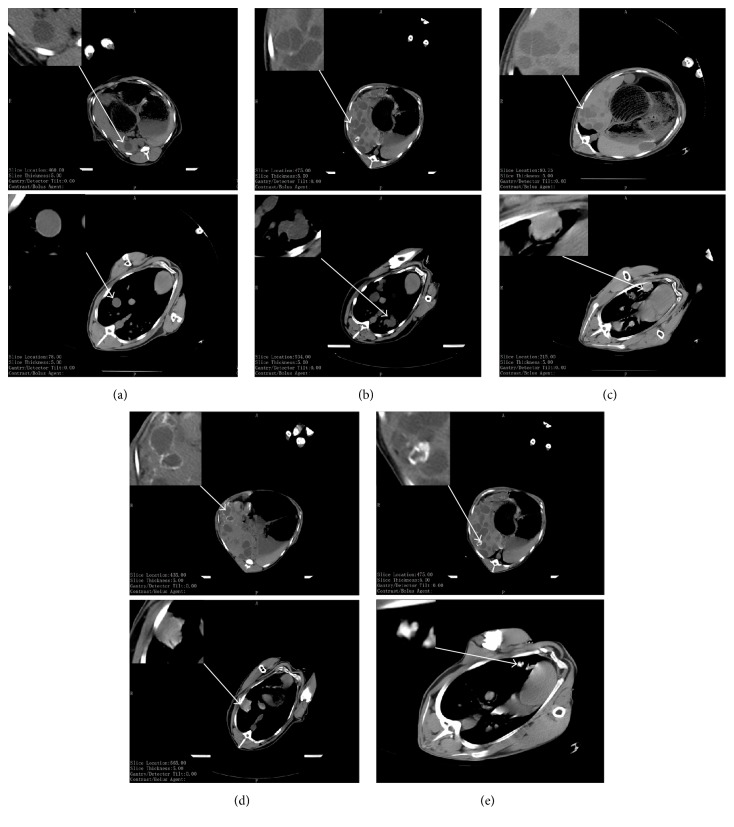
Imaging features of cystic echinococcocal cysts detected by CT. (a) CE1 was in a normally round or oval shape, with a size range of 1–10 cm. (b) Typical image of CE2 with “cyst in cyst,” “honeycomb-like,” or “rose petal” structures. (c) A typical image of CE3 with hydatid cyst wall turning thick and rough. The density of the cyst fluid increased and even showed a pot of calcification. (d) A typical image of CE4 with calcification in the partial cysts. (e) Image of CE5 with a thick calcified wall in the cyst.
